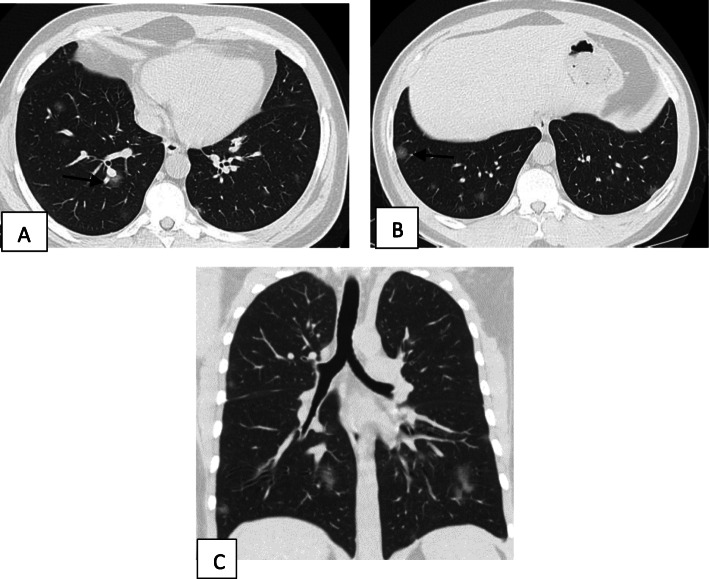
Unenhanced chest CT of a 33-year-old male patient with COVID-19. a, b Axial lung window images show bilateral subpleural and broncho-vascular areas of ground glassing opacity [arrows]. c Coronal reformatted images. CT score is 5
